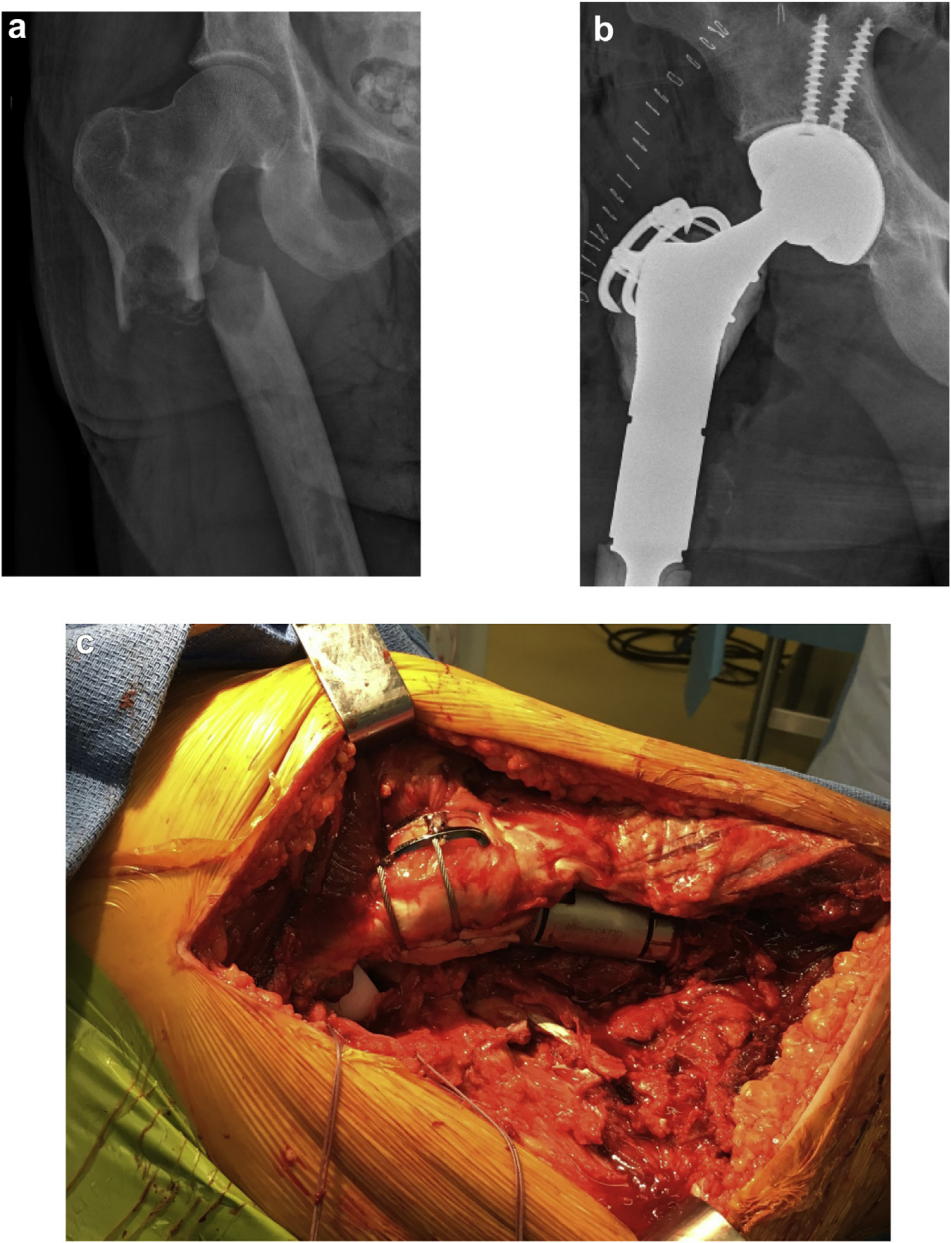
Preoperative (a) and immediate postoperative (b) radiographs. Intraoperative photograph at completion of cemented trochanteric fixation technique in a proximal femoral replacement (c).
